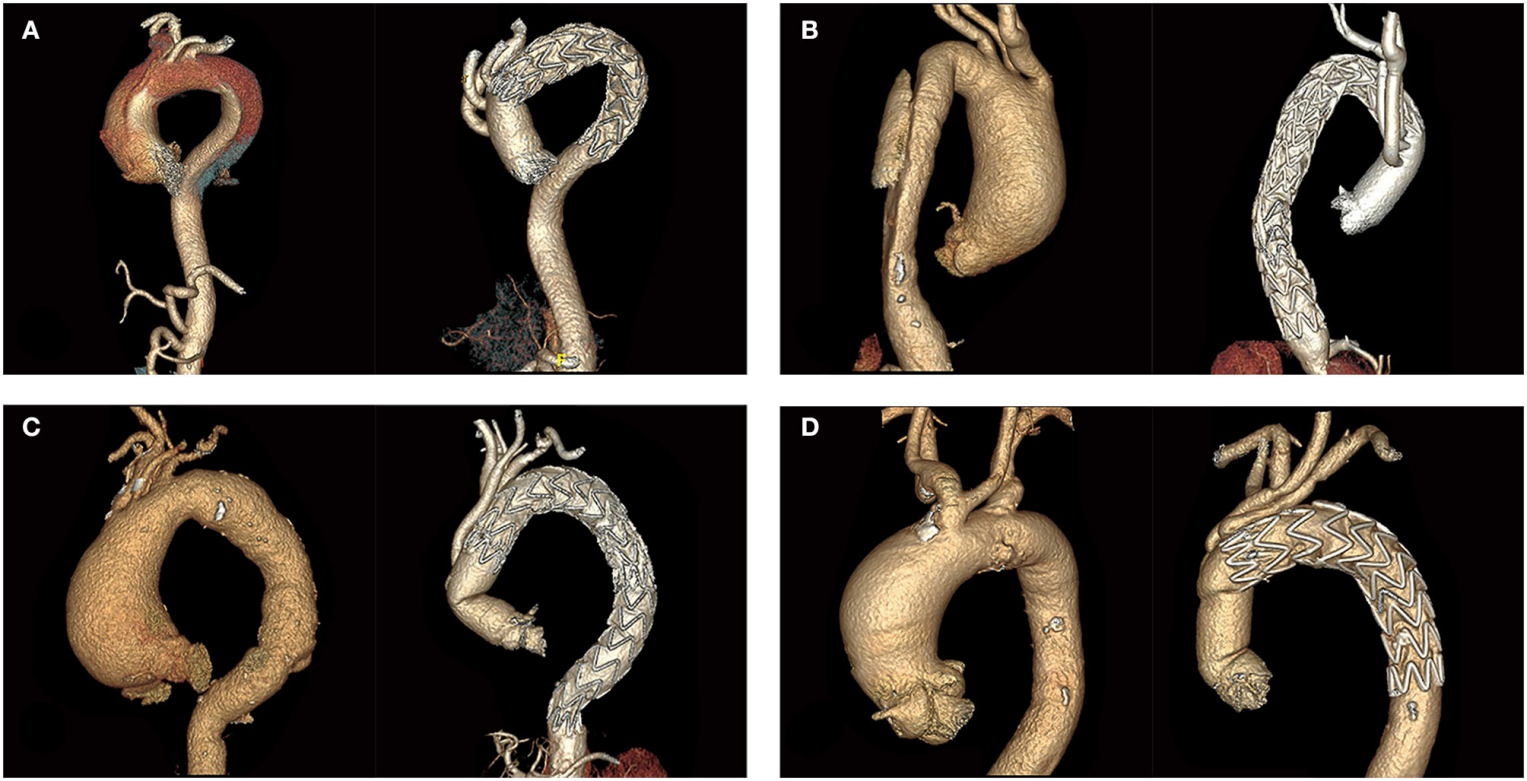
Preoperative and postoperative CT scans of different aortic arch diseases. (A) Acute type A aortic dissection. (B) Acute type B aortic dissection. (C) Aortic arch aneurysm. (D) Penetrating arch ulcer.
